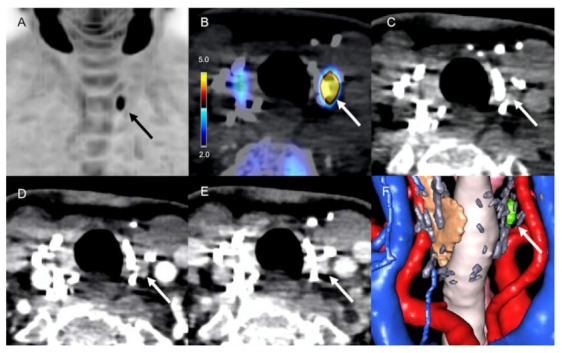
^18^F-FCH PET/CT, 4D-CT and 3D-virtual neck exploration of a 74-year-old woman with recurrent pHPT and a history of left thyroidectomy and removal of the upper right hyperplasic parathyroid gland. From left to right, top to bottom: lower left adenoma (black and white arrows) with high focal accumulation of 18F-FCH ((A) anterior view of maximum intensity projection, (B) axial PET/CT image, SUVmax = 9.67), spontaneous density of 71 HU (C) no contrast enhancement on arterial-phase CT ((D) density of 71 HU), delayed enhancement at the late venous phase ((E) density of 140 HU), 3D-virtual neck exploration (F).
